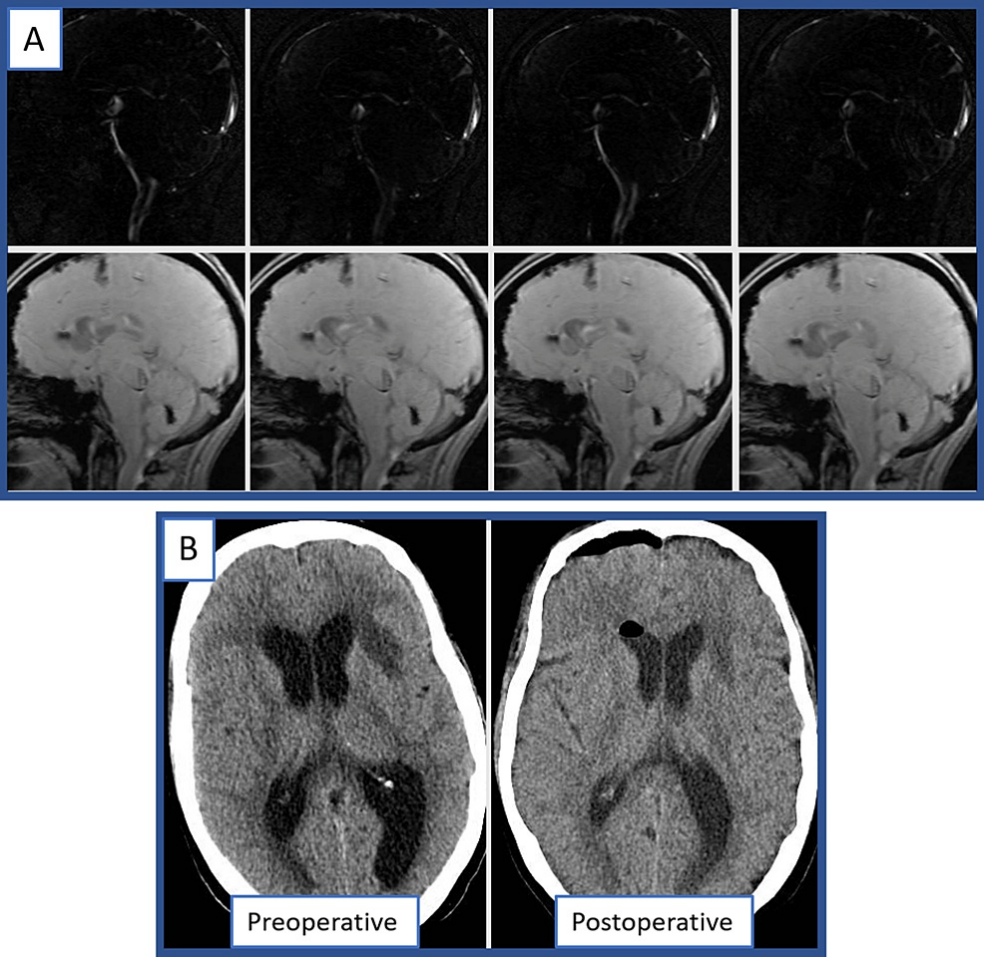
Postoperative imaging MRI flow study postoperatively demonstrated flow through the third ventricular floor fenestration (A), with a reduction in hydrocephalus visible on MRI as well as postoperative CT scans (B). This figure was prepared for this submission using original images, and no credit/license is required.
